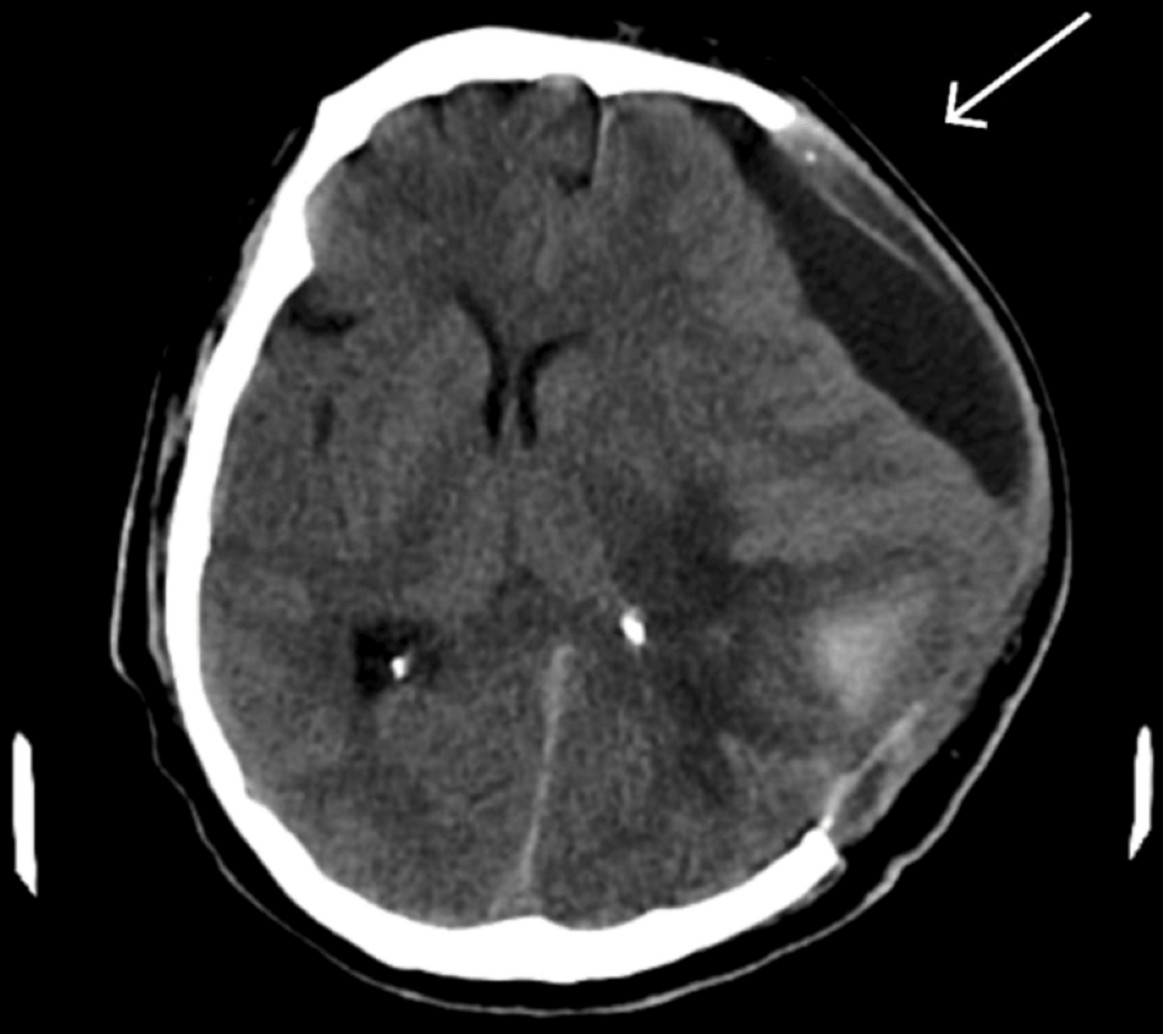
CT brain, with the arrow pointing towards hygroma.
